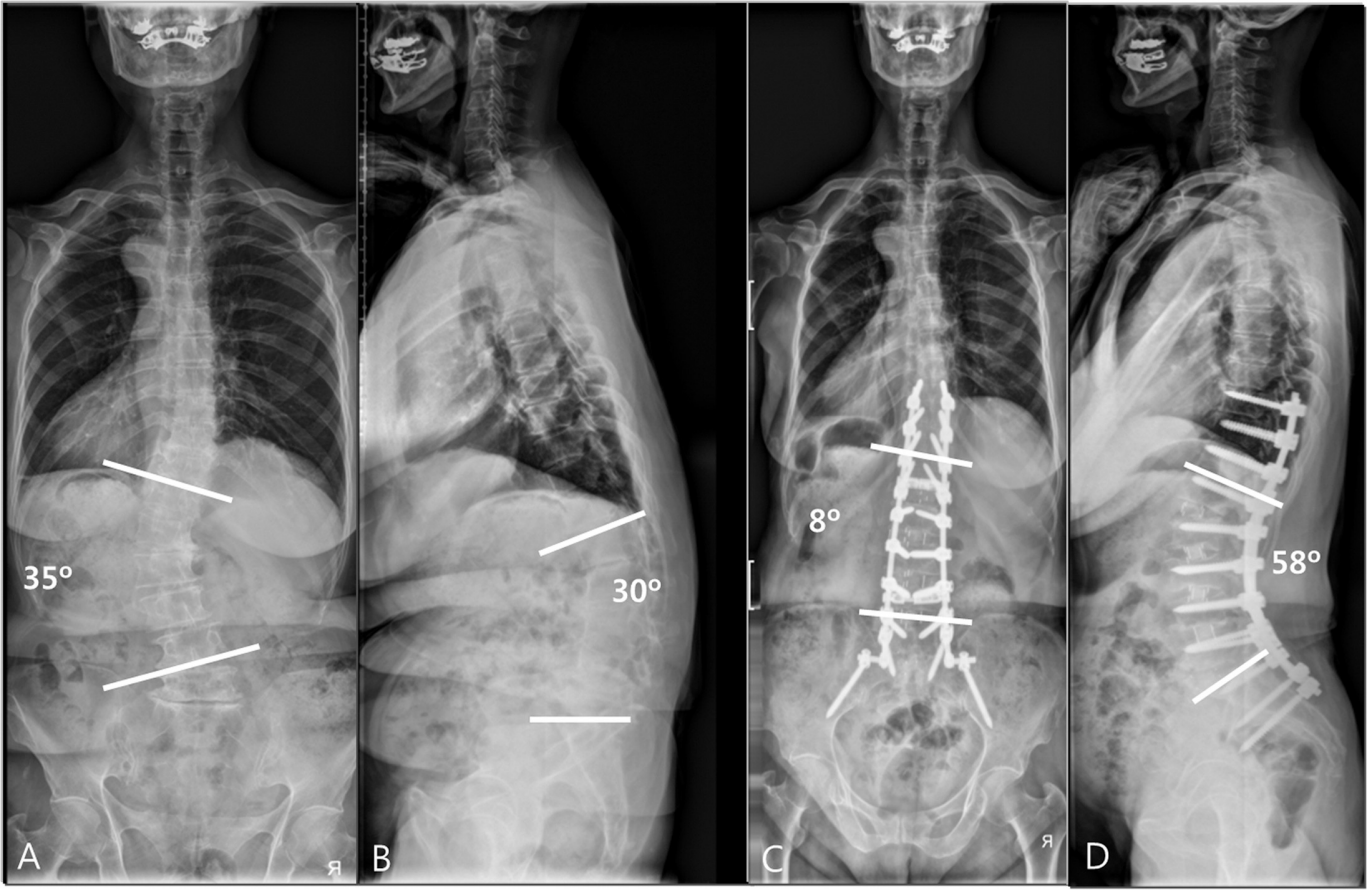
(A, B) Anteroposterior (AP) and lateral standing radiographs of a 70–year–old woman who had severe back pain and pain in both legs, showing degenerative lumbar kyphoscoliosis. A three–step (posterior–oblique–posterior) approach was used to obtain adequate sagittal and coronal balance. (C, D) Postoperative AP and lateral radiographs showed L1–5 interbody fusion and good sagittal alignment.
